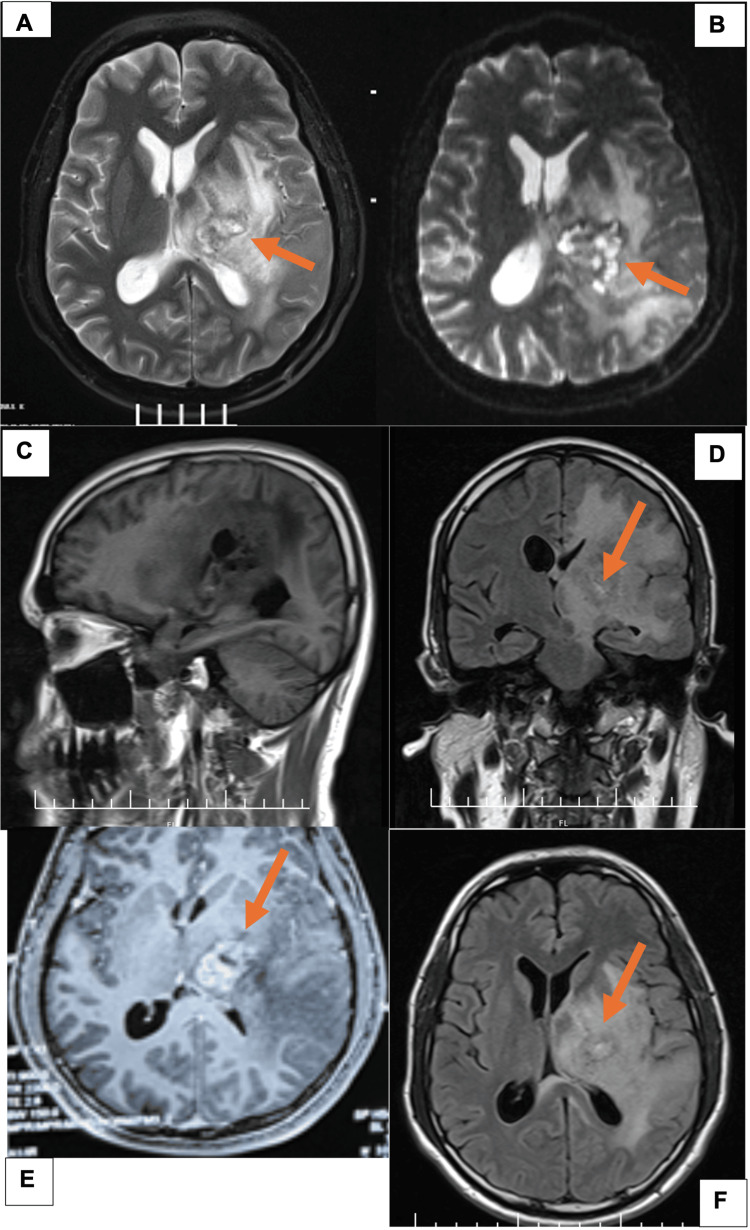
Preoperative MRI imaging Preoperative T2-weighted axial images (A & B), T1-weighted sagittal image (C), fluid-attenuated inversion recovery (FLAIR) coronal image (D), T1 post-contrast axial image (E), and FLAIR axial image (F).
